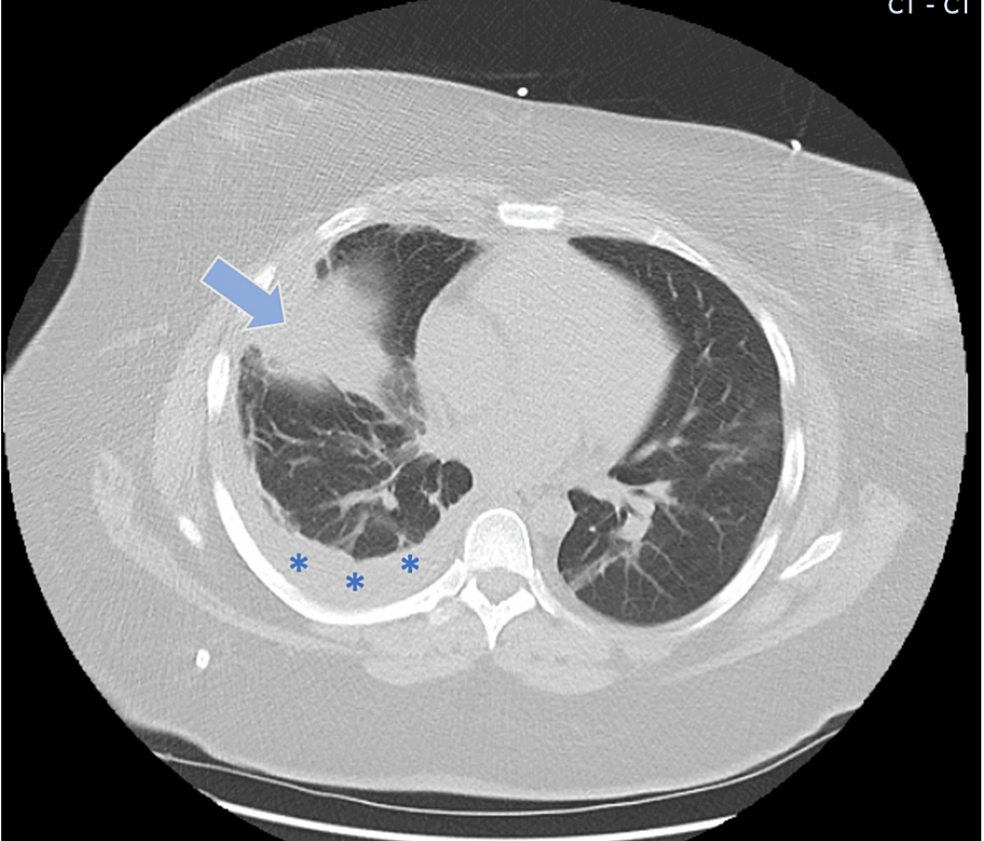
Chest CT findings Chest CT with right middle lobe infiltrate (blue arrow) redemonstrated, also confirming the presence of pleural effusion on the right side (asterisks) CT: computed tomography
